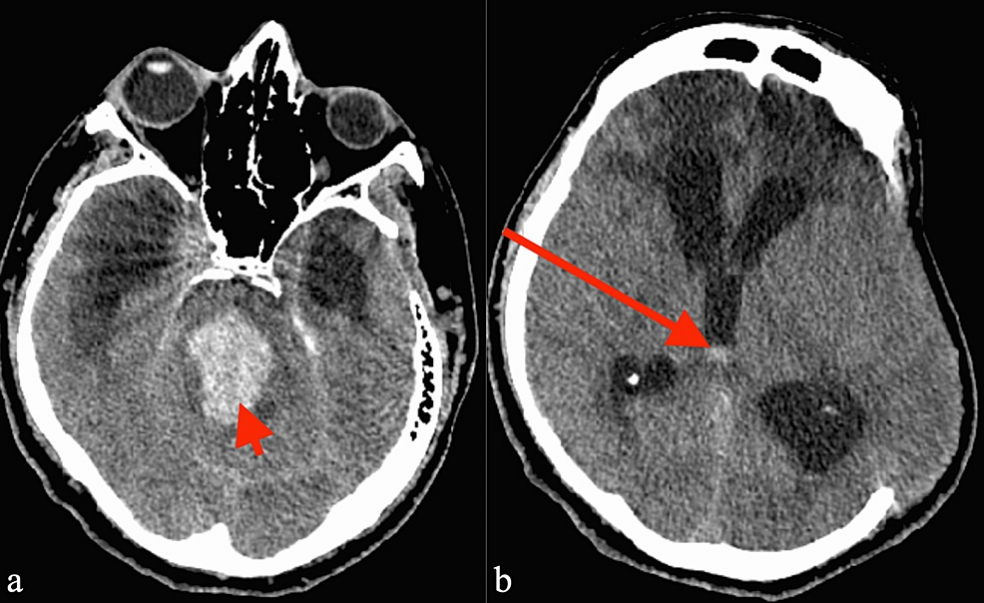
Axial CT head five days after lumbar drain demonstrating (a) catastrophic brainstem hemorrhage encompassing fourth ventricle (short red arrow) and (b) a mild decrease in the ventricle size and amount of brain parenchyma protruding through craniectomy skull defect, with intraventricular hemorrhage in the third ventricle (long red arrow)
